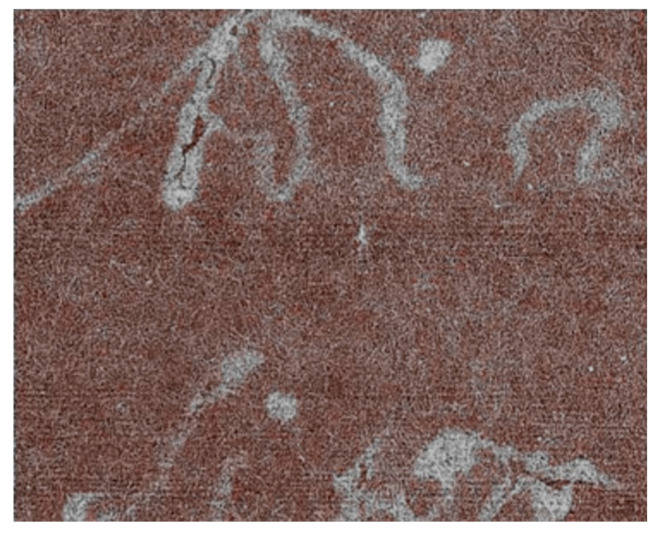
Radiography reads ancient handwritings with “iron gall” inks without requiring the opening of books [29,30,31].
